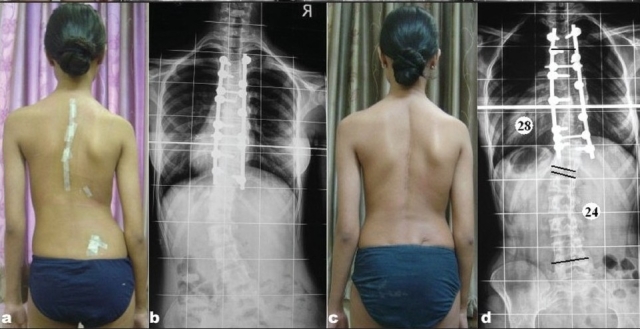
Immediate postoperative clinical picture (a) and anteroposterior radiograph (b) depicting slight decompensation. 6 months follow up clinical picture (c) and radiograph (d) showing restored coronal spinal balance with final Cobb's angles; thoracic 28°, Lumbar 24°
